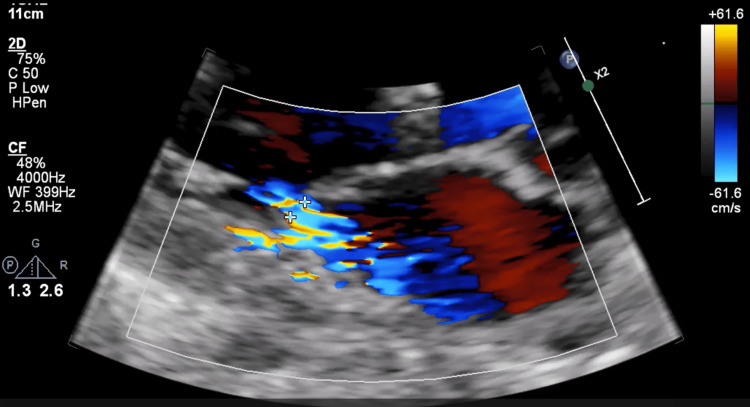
Parasternal long axis-view showing upper mild mitral regurgitation
